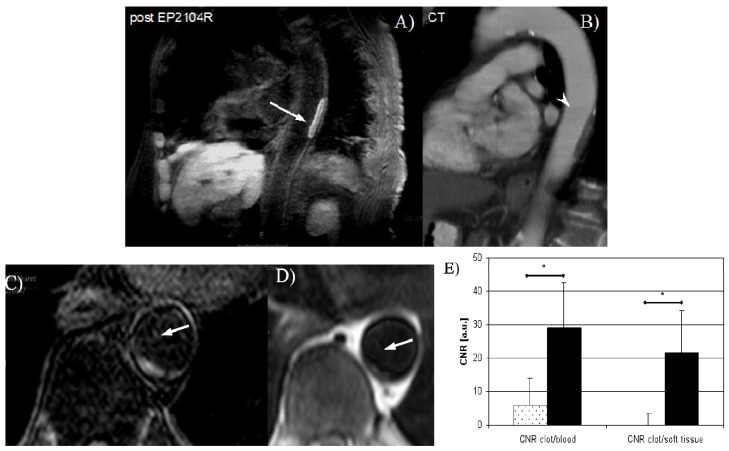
Human thrombus imaging using EP-2104R, a fibrin binding contrast agent, in a patient with aortic thrombus. A mural aortic thrombus is well delineated on inplane post EP-2104R black blood MR images (A) while differentiation between thrombus and vessel wall is poor on multi slice CT images (B). Excellent aortic thrombus visualization on cross-sectional delayed enhancement images post EP-2104R (C) while differentiation between thrombus and vessel wall is poor on pre contrast black blood turbo spin echo (D) or gradient echo (E) MR images [117].
